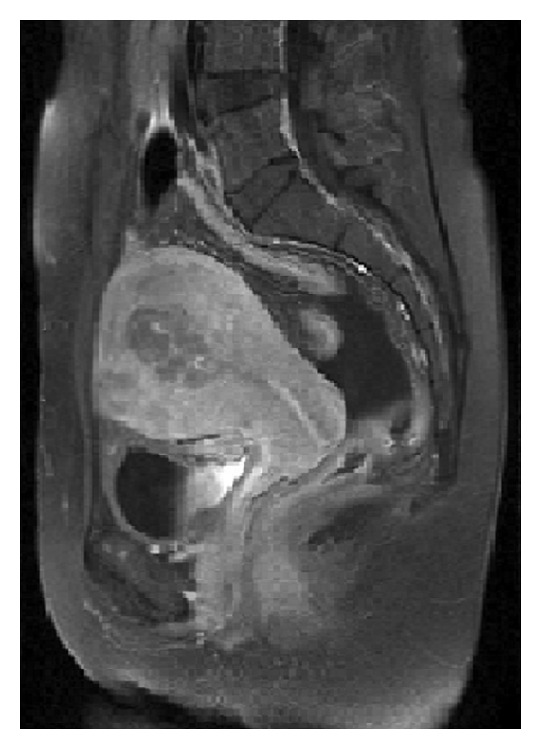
Pelvic MRI. Increase in diffuse thickness of uterus.
